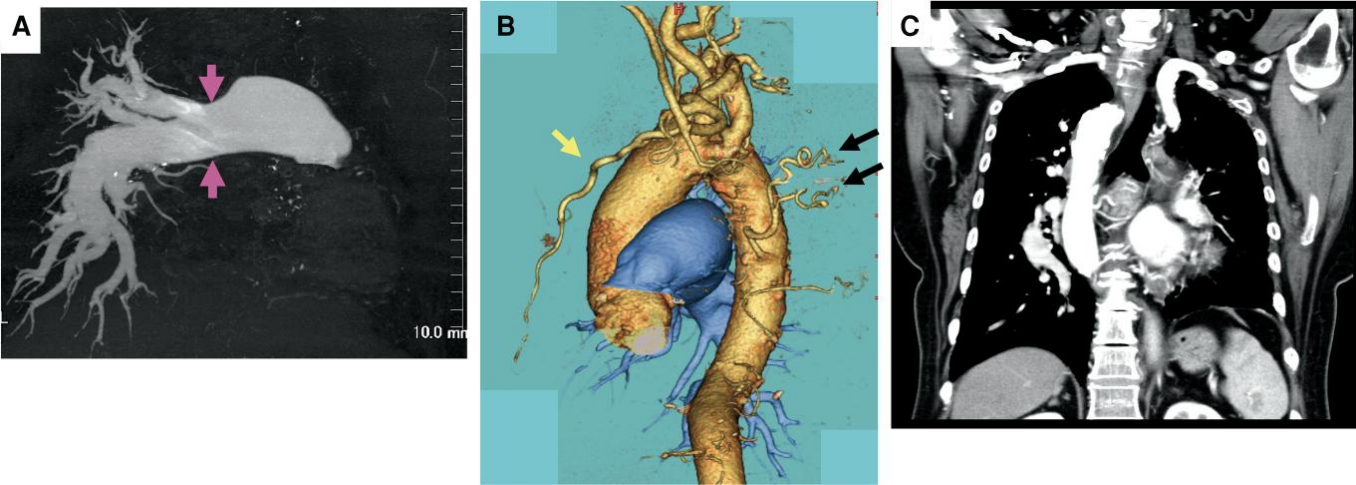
(*A*) Three-dimensional CT scan of the chest showing dilatation of the main trunk of the right pulmonary artery (arrow) and a defect from the origin of the left pulmonary artery. The right pulmonary artery is tapered peripherally but is not occluded. (*B*) The left internal thoracic artery (arrows) and left bronchial arteries (arrows) are well developed to supply blood flow to the left lung. (*C*) Coronal section of enhanced CT scan showing the right aortic arch. CT, computed tomography.
